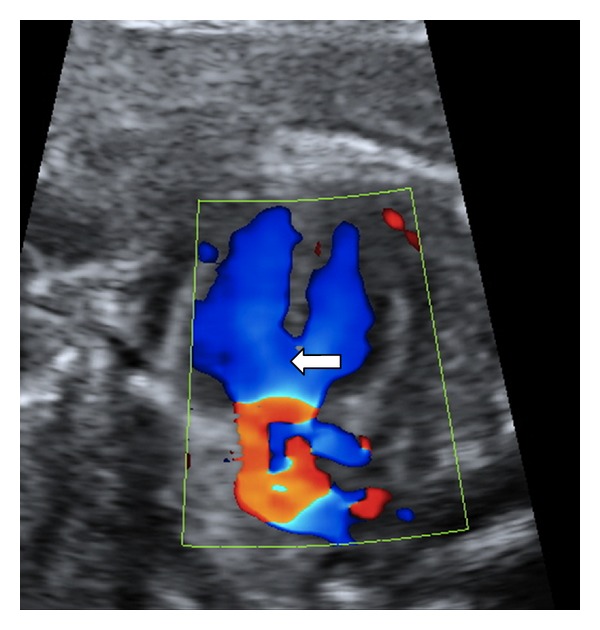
Color Doppler ultrasound showing the “Y sign”. Blood flow passes from the right and left ventricles, through the VSD (arrow), into an overriding enlarged aorta. Turbulent flow is shown as reddish areas around the aortic valve.
